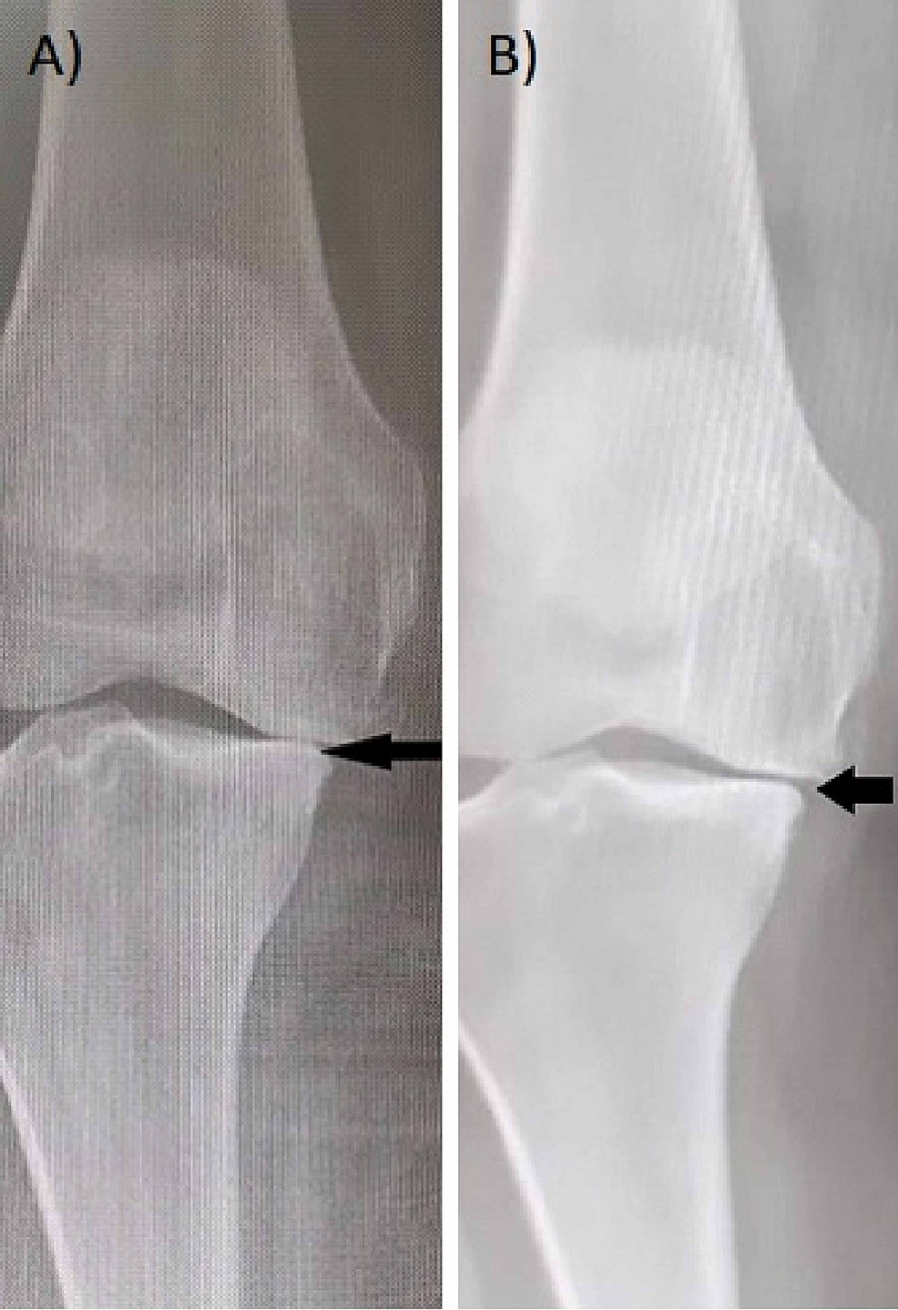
X-rays weight bearing view for the same patient almost one year apart: A) On 16/05/2018 and showing loss of joint space (arrow). B) On 26/04/2019 after treatment of four Platelet Rich Plasma injections showing improvement in joint space (arrow) denote starting cartilage formation.
